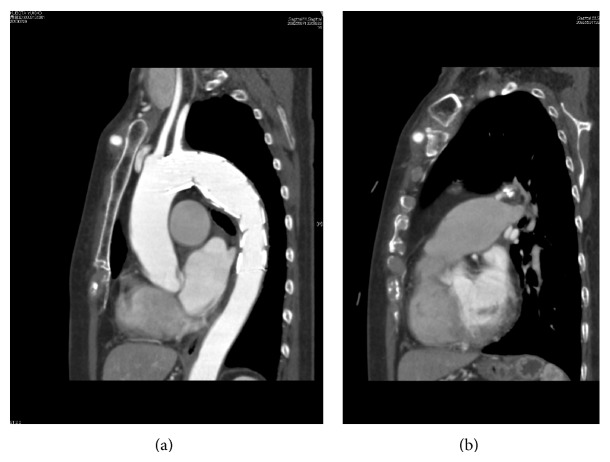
(a) A curved planar CT reformation image showing the TAA with stent grafts. (b) A curved planar reformation CT image showing the PDA without contrast enhancement. CT, computed tomography; PDA, patent ductus arteriosus; TAA, thoracic aortic aneurysm; LAO, left anterior oblique.
